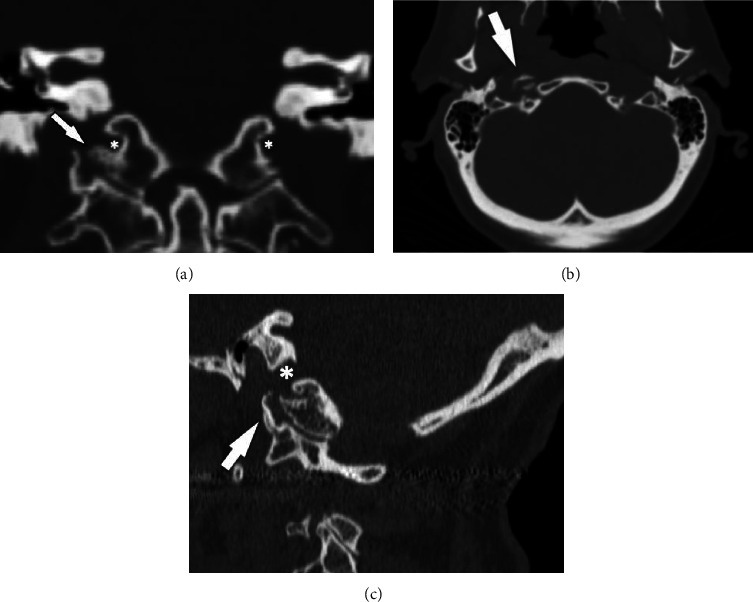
(a) Coronal reformatted CT image shows osteophytosis (arrow) of the atlanto-occipital articulation on the right. The osteophyte arising from the occipital condyle narrows the hypoglossal canal (asterisks). (b) Axial CT showing osteophyte in the right hypoglossal canal. (c) Sagittal CT showing osteophyte in the right hypoglossal canal.
